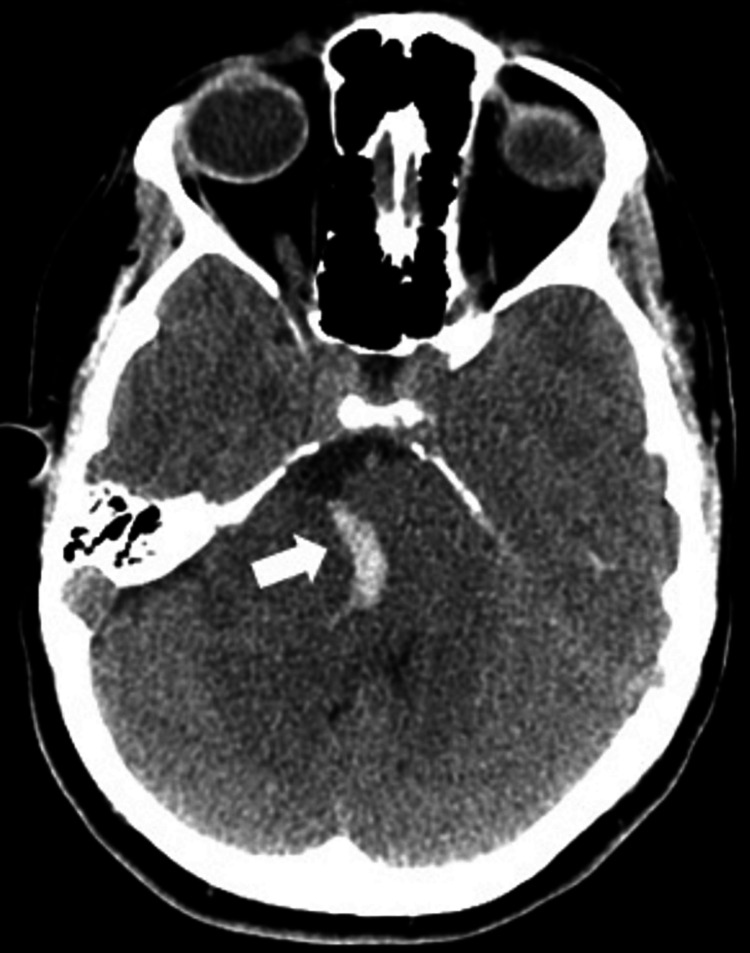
Axial pre-operative CT scan. Axial brain CT scan slice revealing spontaneous hyperdensity in the posterior fossa, suggestive of acute hemorrhage. Marked deformation of the brainstem, middle cerebral peduncle and cerebellum, with shift to the left and near collapse of the fourth ventricle.
